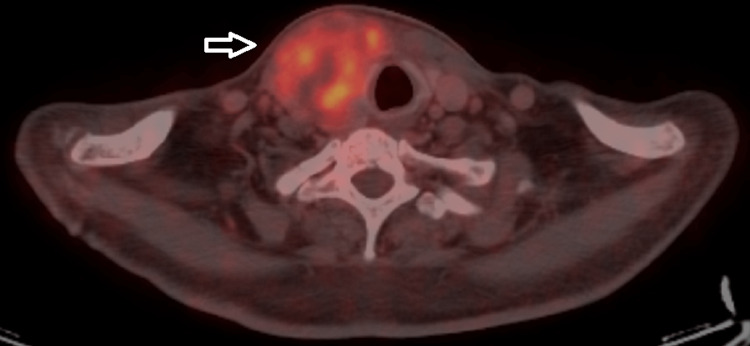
FDG-PET CT showing hypermetabolic heterogeneously enhancing mass lesion in the right lobe of the thyroid (arrow) FDG: fluorodeoxyglucose, PET: positron emission tomography.
